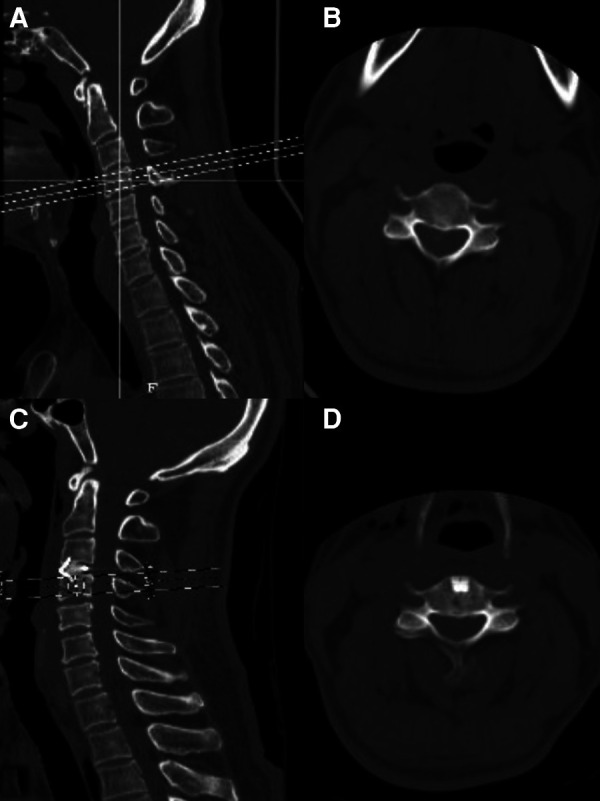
(A) Preoperative C3/4 CT sagittal plane. (B) Preoperative C3/4 CT cross-section. (C) Postoperative C3/4 CT sagittal plane. (D) Postoperative C3/4 CT cross-section.
